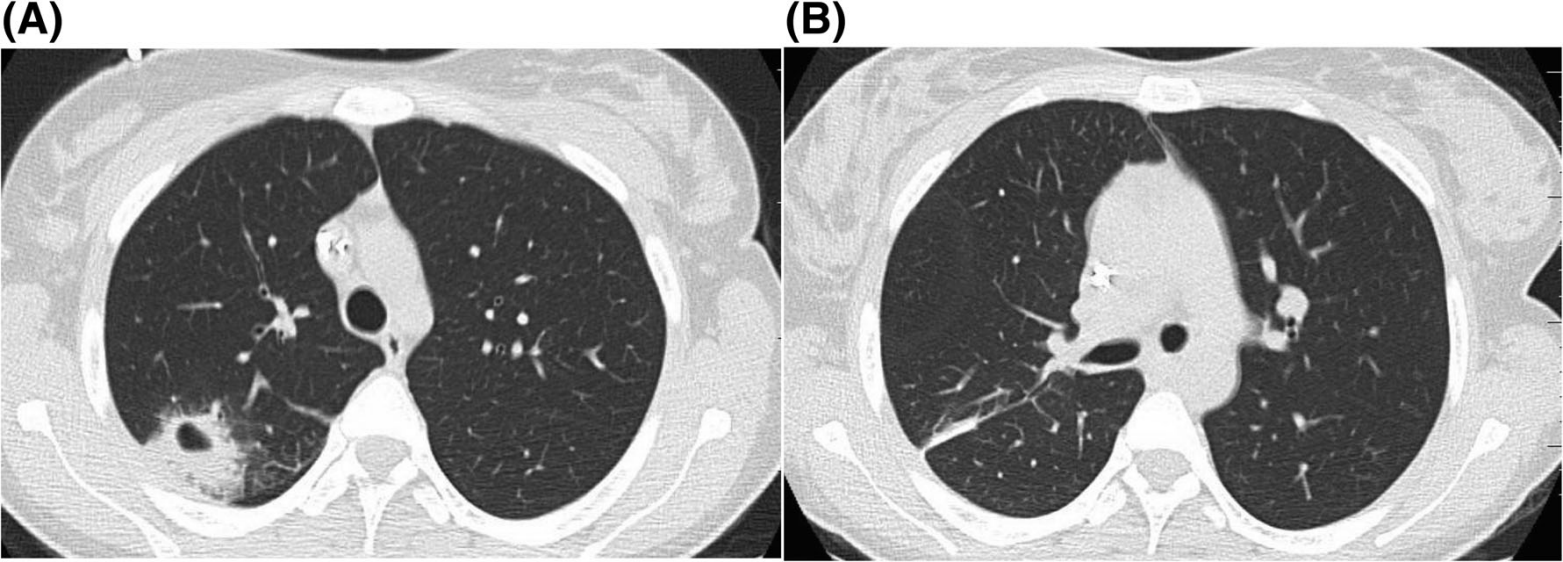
CT scans of the chest showing on the left. A, Preinduction myeloid sarcoma shown as a cavitary lesion with surrounding consolidation and ground glass; and on the right. B, A postoperative CT scan of the chest after wedge excision of the remnant lesion postinduction
